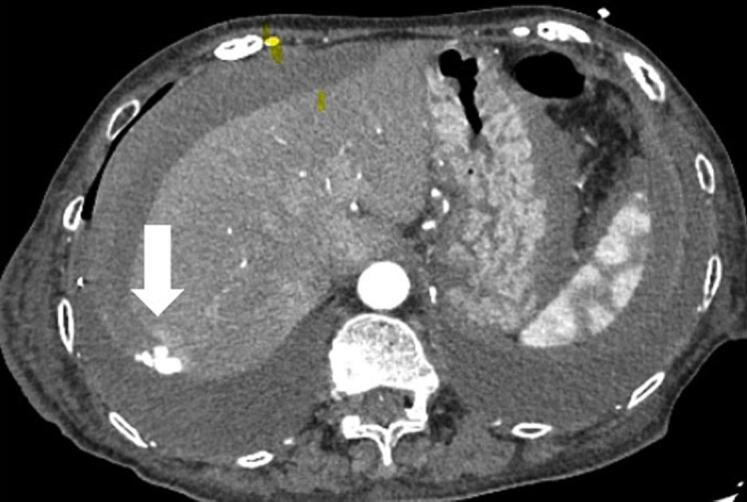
Computed tomography shows extravasation from the right inferior phrenic artery (arrow).
